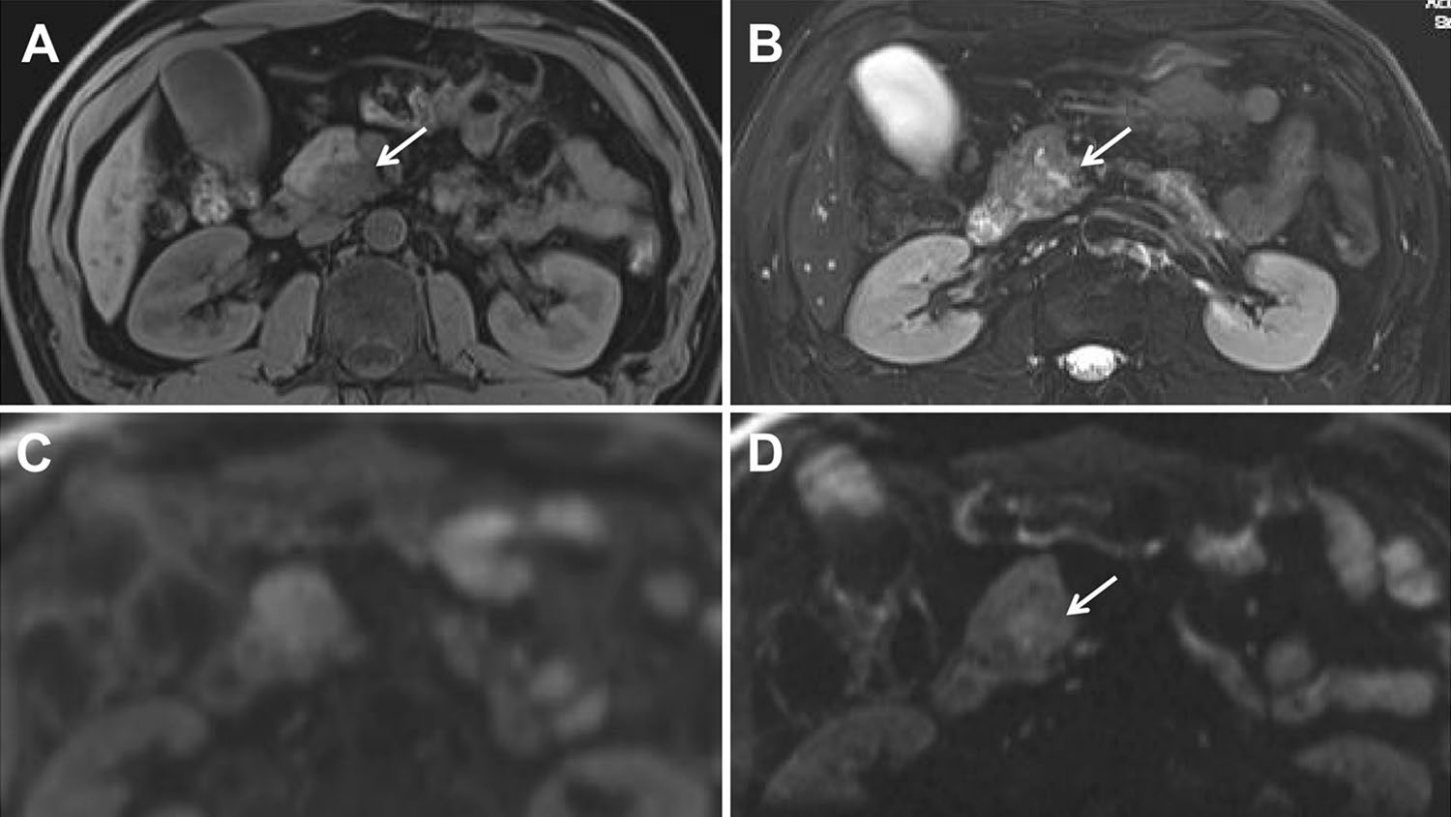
A 42-year-old man with pancreatic head carcinoma with a diameter of 19.0mm. A T1-weighted MR image and B T2-weighted MR image show a mass (arrow) in the pancreatic head. C c-EPI DWI shows no abnormality in the periampullary region D z-EPI DWI shows increased signal intensity of the lesion with better delineation and clear margin (arrow)
